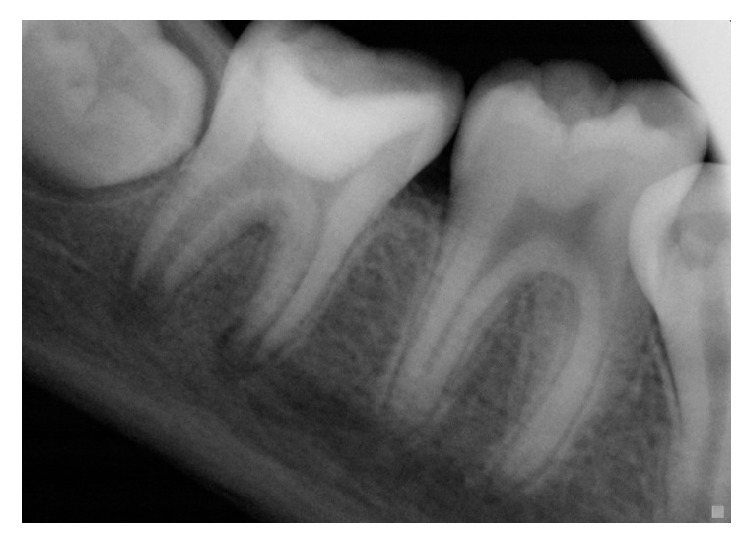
Initial radiography: tooth 47 with radiolucent images at the mesial and distal roots.
